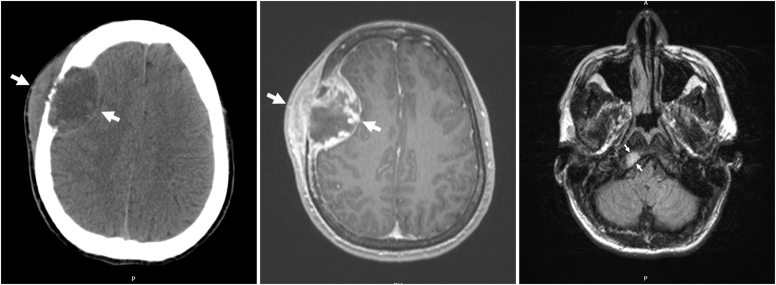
Imaging findings on presentation. (Left) Computed tomography of the brain without contrast showed a destructive lesion (wide arrows) centered in the right frontal bone with associated intracranial and extracranial extensions. The intracranial dural-based component measured 4.9 × 3.2 cm, and the extracranial component measured 4.9 × 1.3 cm. There was a leftward midline shift of 6 mm due to mass effect. (Middle) Magnetic resonance imaging with gadolinium confirmed the right frontal lesion (wide arrows), which exhibited intense enhancement. (Right) Magnetic resonance imaging T2 fluid-attenuated inversion recovery protocol revealed a second lesion in the right occipital bone, which measured 1.5 cm (thin arrows).
